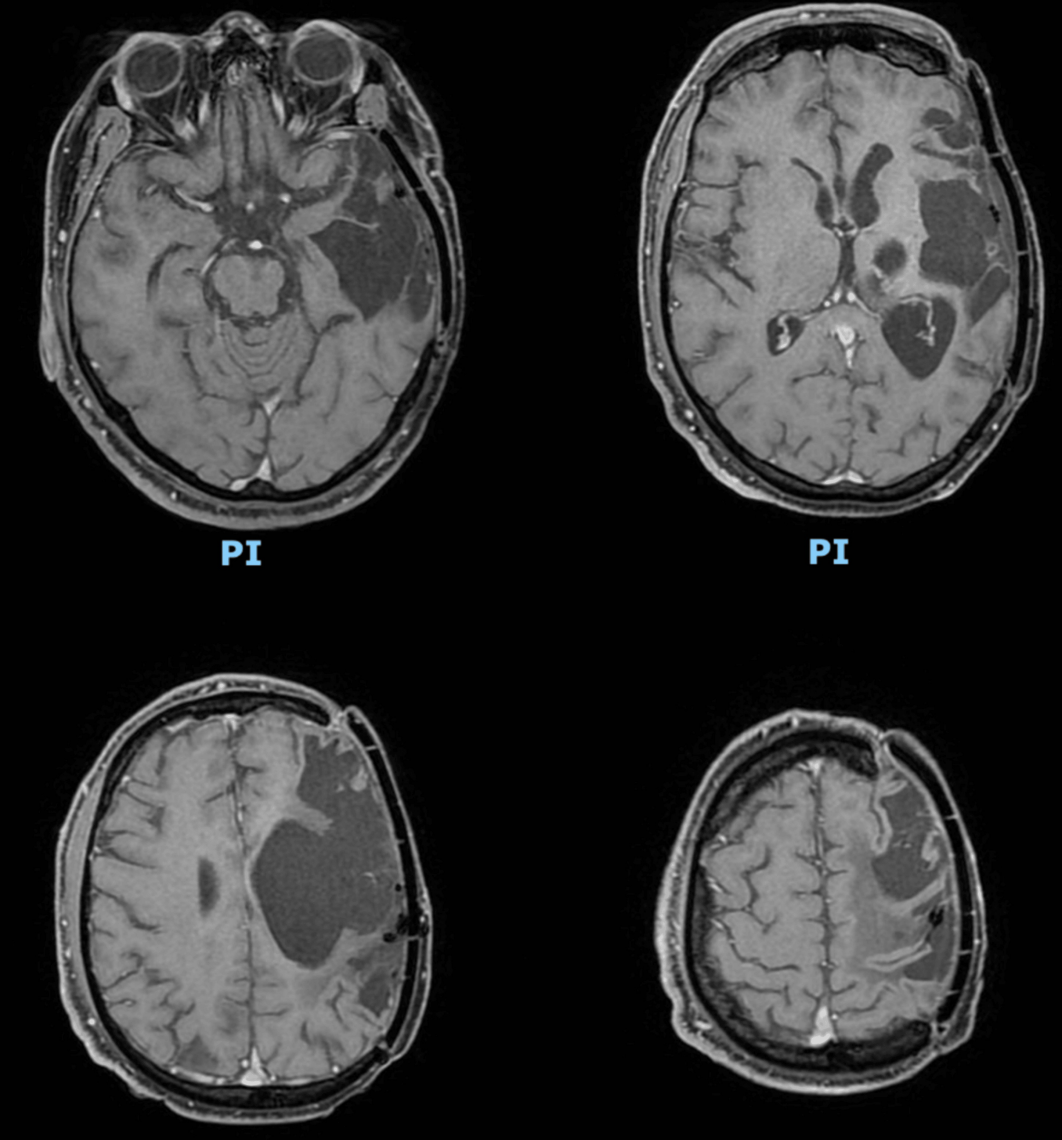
Postoperative contrast-enhanced MRI, 2024 In the axial section, no recurrent lesion is observed, and an area of encephalomalacia is present at the site of surgical resection.
